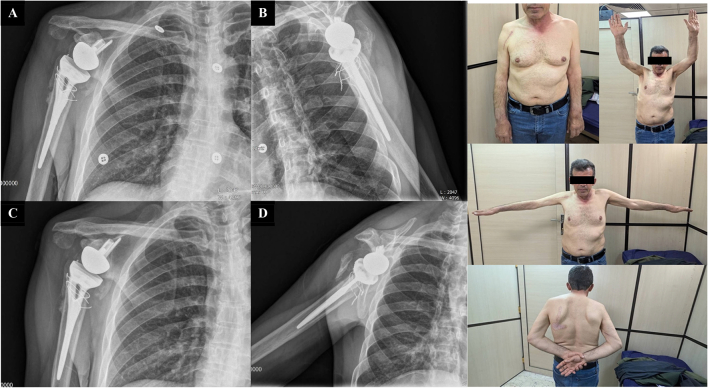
Serial follow-up radiographs following the reverse shoulder arthroplasty, at 3-month (A) and (B) and 6-month (C) and (D) follow-up, with no signs of prosthetic dislocation, loosening, or acromial/periprosthetic fractures. At 6-month follow-up Clinical photographs demonstrate that the patient has regained near-complete range of motion.
